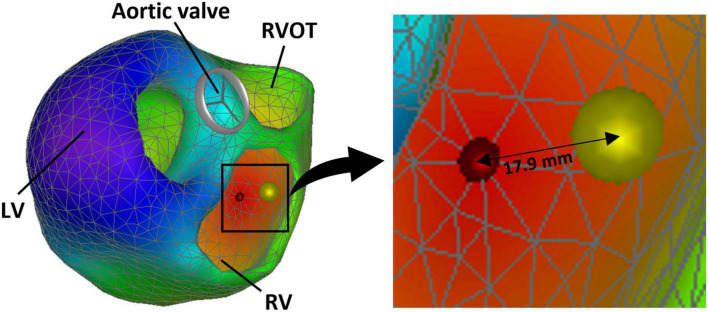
Reconstructed 3D ventricular electrical activation sequence showing the reconstructed initial site of activation (rISA) (red marker) and the right ventricular (RV) lead position assessed from computed tomography (CT) (yellow marker). The localization error (LE) is the geodesic distance between the center of the CT marker and the estimated initial site of activation. LV, left ventricle; RVOT, right ventricular outflow tract.
